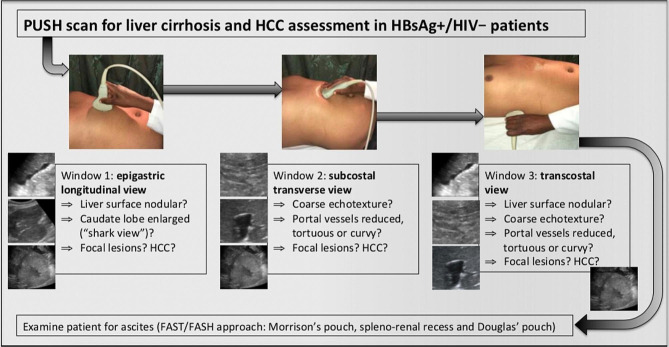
Point-of-care UltraSound for hepatitis - the PUSH protocol
